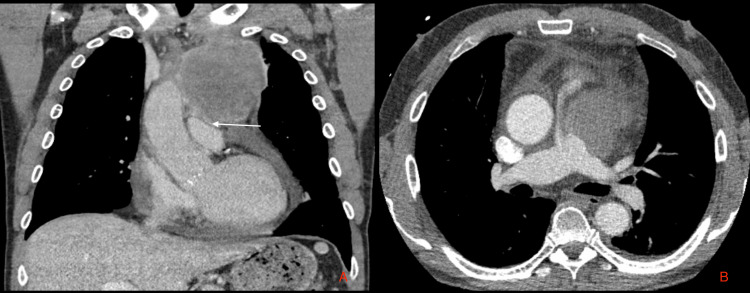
Chest CT of the patient A. Intravenous contrast coronal view showing a 10-cm anterior mediastinal mass with pericardial invasion (white arrow). B. Transverse view showing partially compressed main pulmonary artery by irregular mediastinal mass CT: computed tomography
